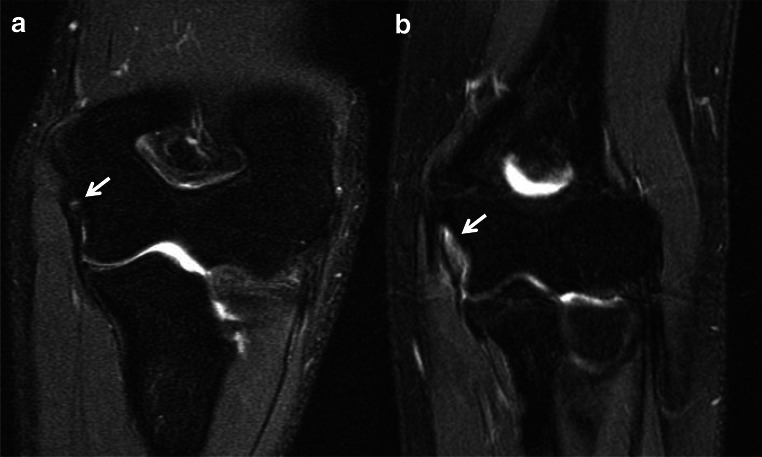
Coronal T2 MR images of the elbow in different patients demonstrate a low- (a, arrow) and high-grade (b, arrow) partial thickness tears of the UCL
